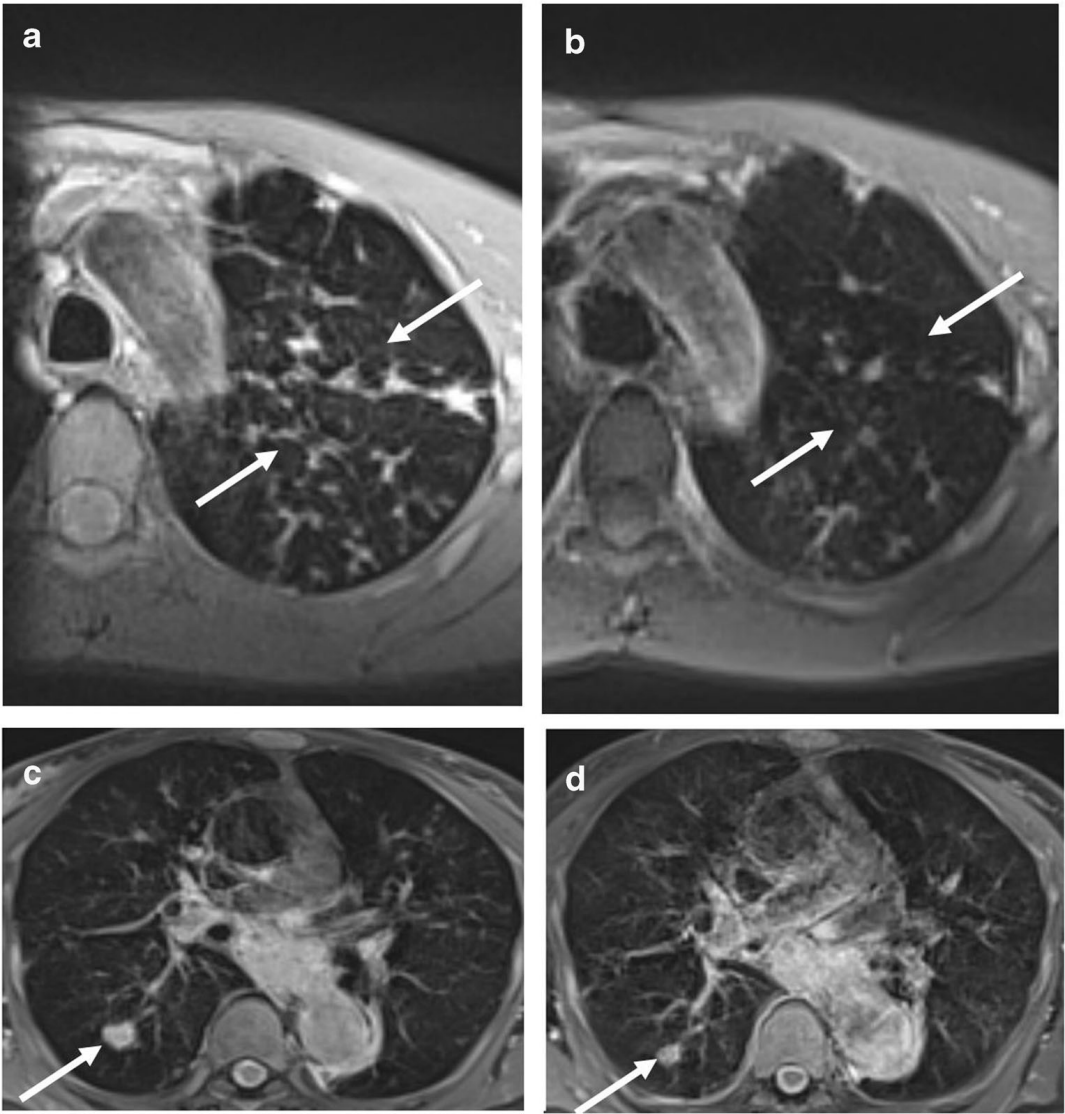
Representative MRI sequences in a patient at diagnosis and after 12 months In a 18 years old man who started Ig replacement therapy (patient n. 4), MRI BLADE sequence at first MRI showed multiple distal mucus plugs with branching patterns (“tree in bud” appearance) of the left upper lobe (*white arrows*) (a); these abnormalities regressed at the second MRI assessment after 12 months (*white arrows*) (b). MRI BLADE sequence at first MRI showed one nodule (*white arrow*) at the right lower lobe (c); the nodule showed a reduction in size at the second MRI assessment after 12 months (white arrow) (d)
